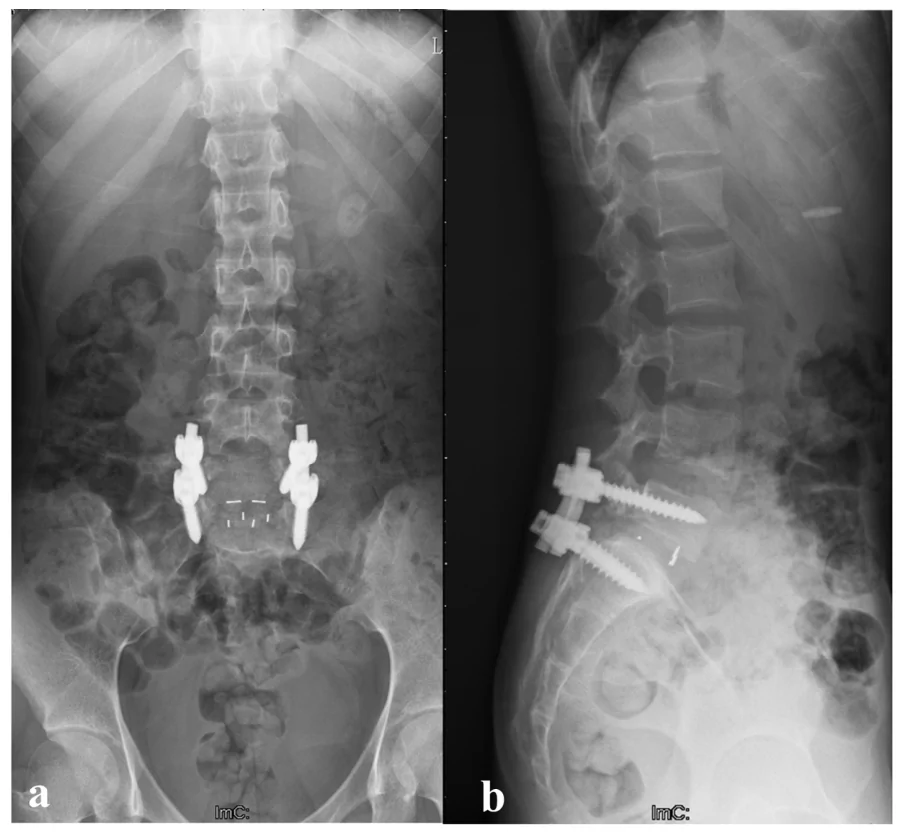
Postoperative anteroposterior (a) and lateral (b) radiographs showing L5-S1 posterior instrumentation and interbody fusion with restoration of lumbosacral alignment.
